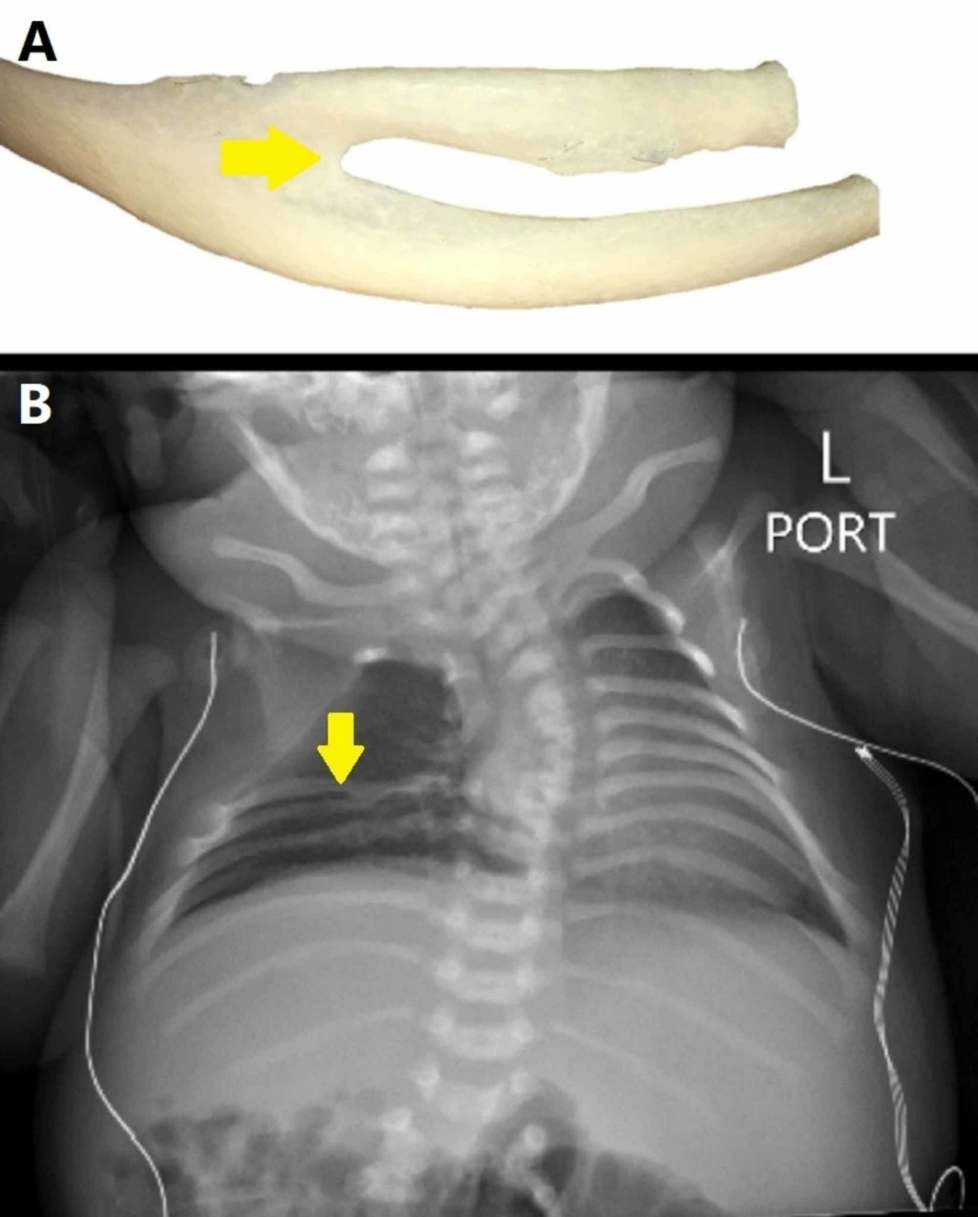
Bifid rib (A) Closer lateral view of a right bifid rib. Note the bifurcation before attaching to the sternum (yellow arrow). (B) Radiograph of a child with with right-sided fork-type bifid ribs with mild scoliosis (yellow arrow). Bifid rib, which usually has no associated symptomatology, is a bifurcation of the anterior portion of the rib before fusion with the sternum. There is also bifurcation of the associated neurovascular bundle [8].
